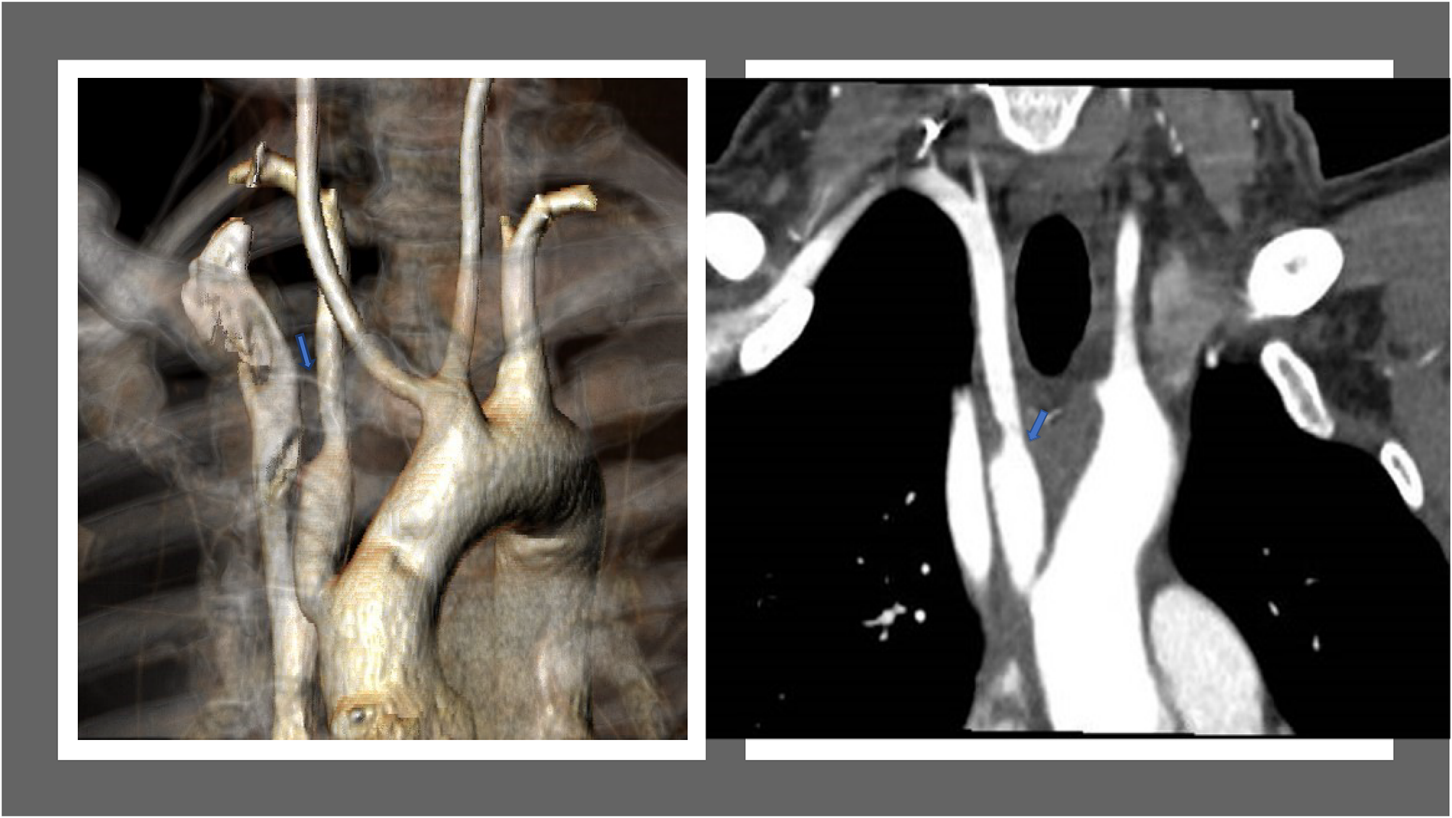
(left)post-operative three-dimensional CT reconstruction imaging depicts the newly established pathway of the right subclavian artery. (right)
